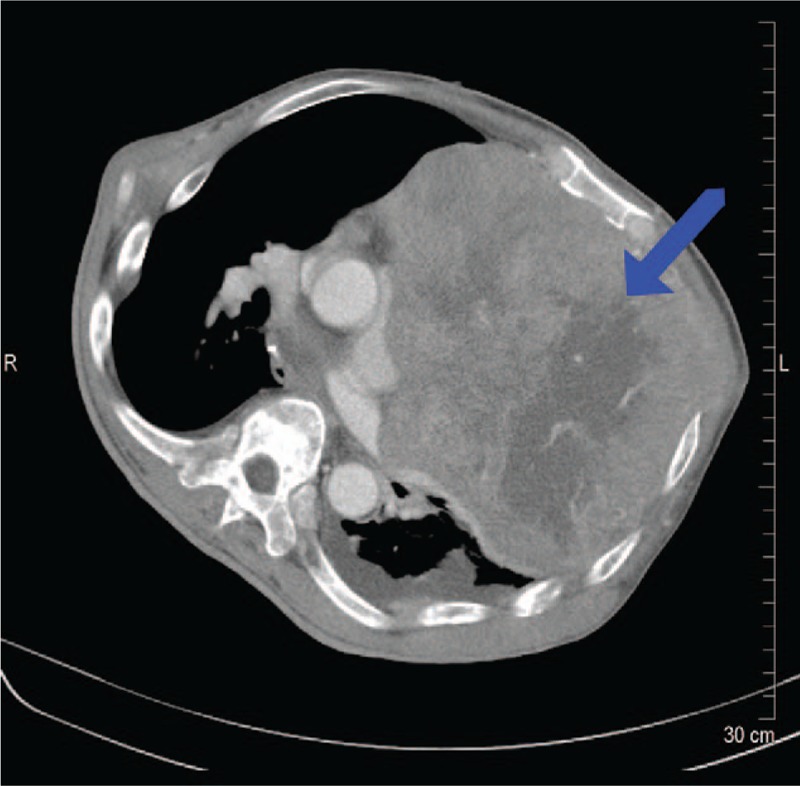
Thoracic computed tomography (CT) scan revealed an anterior mediastinal mass (*arrow*). CT = computed tomography.
